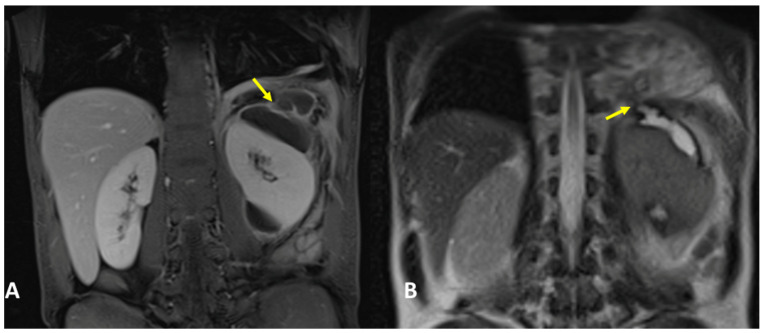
(A,B) Contrast-enhanced MRI, coronal planes, and CE T1- (A) and T2-weighted sequences (B) (same patient as Figure 7). MRI confirmed a left pleural empyema and multiple pancreatic pseudocysts located between the diaphragm, the pancreas tail, and the left kidney. The fistulous tract was detected (arrows).
